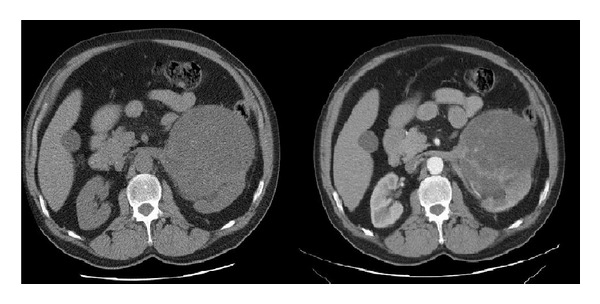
The view of the cystic, thick-walled, septated, and vascularized mass at the arterial phase of the computed tomography.
